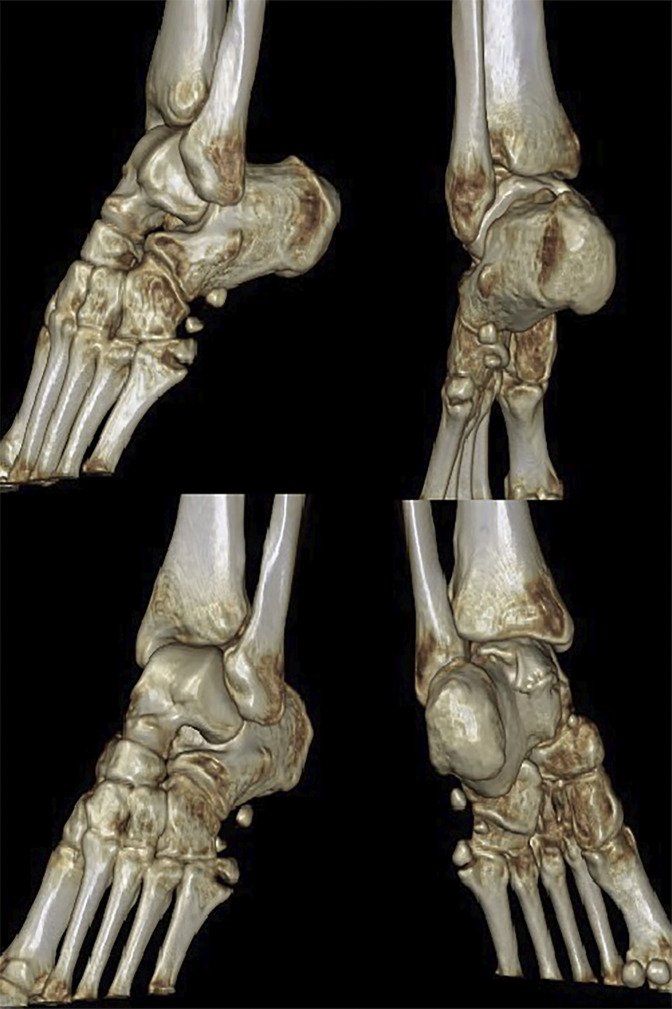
Three dimensional CT scan showing a fifth metatarsal base fracture and displaced os peroneum fracture.
